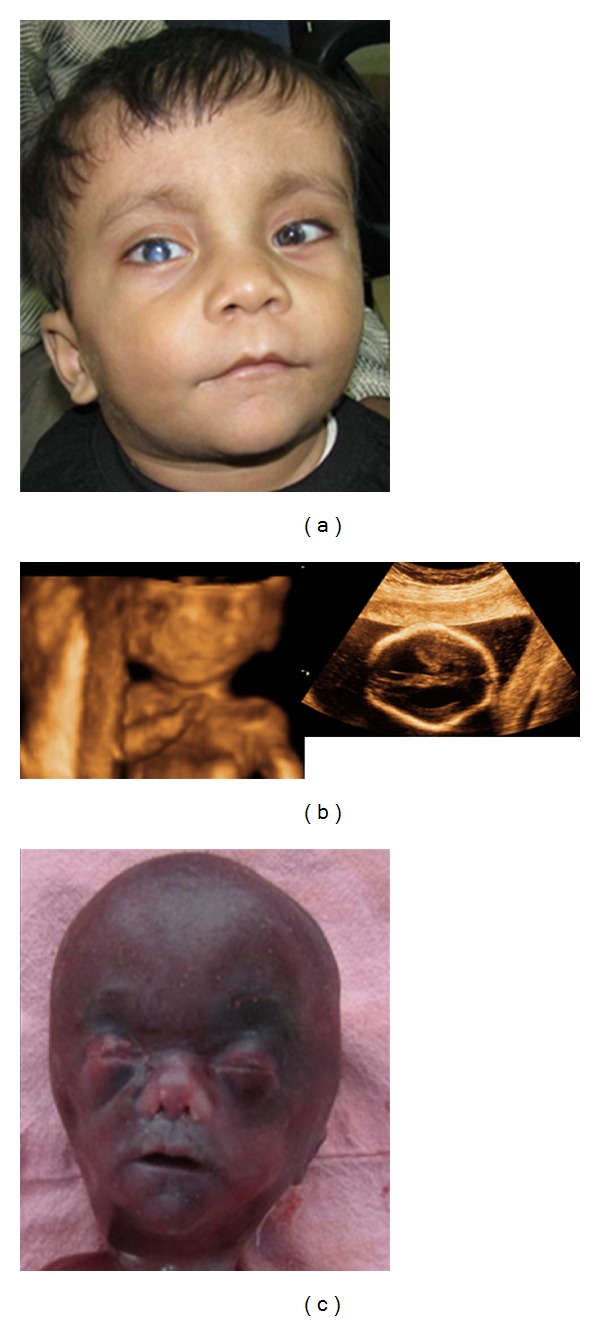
(a) Proband with Peters' anomaly and facial dysmorphism. (b) Ultrasound showing, long philtrum, short nose and ventriculomegaly. (c) Fetus with facial dysmorphism. Note prominent forehead, hypertelorism, long philtrum, short nose, anteverted nostrils, and thin upper lip.
